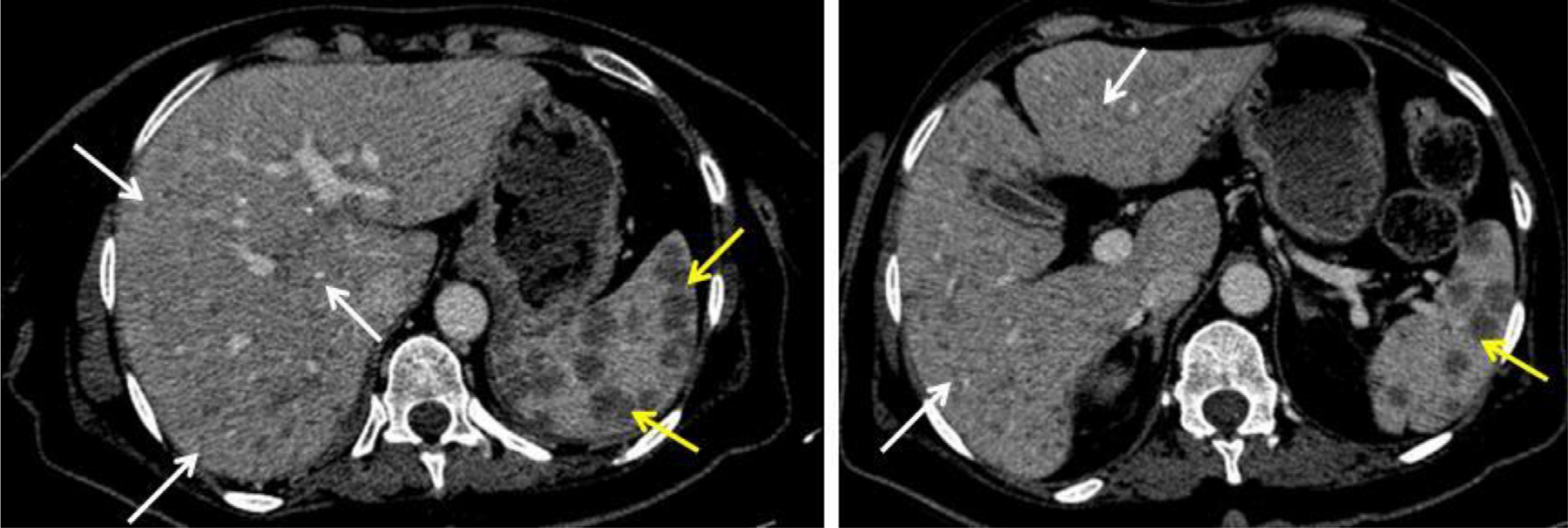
Axial section of abdominal CT scan after injection of contrast medium showing multiple hypodense nodules of the liver sparing no segment (white arrows) and the spleen (yellow arrows).
